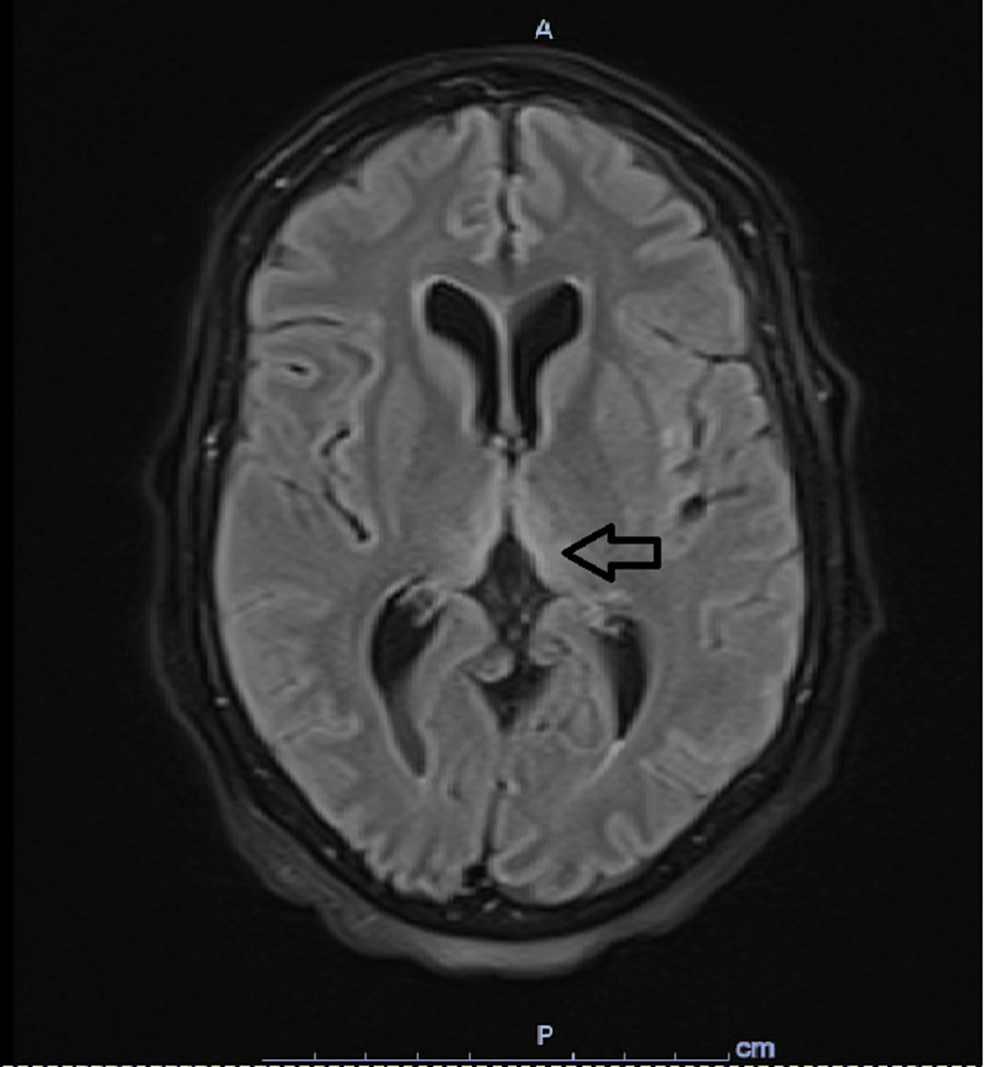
Initial MRI brain MRI of the brain obtained initially showing bilateral thalami hyperintensities (arrow).
